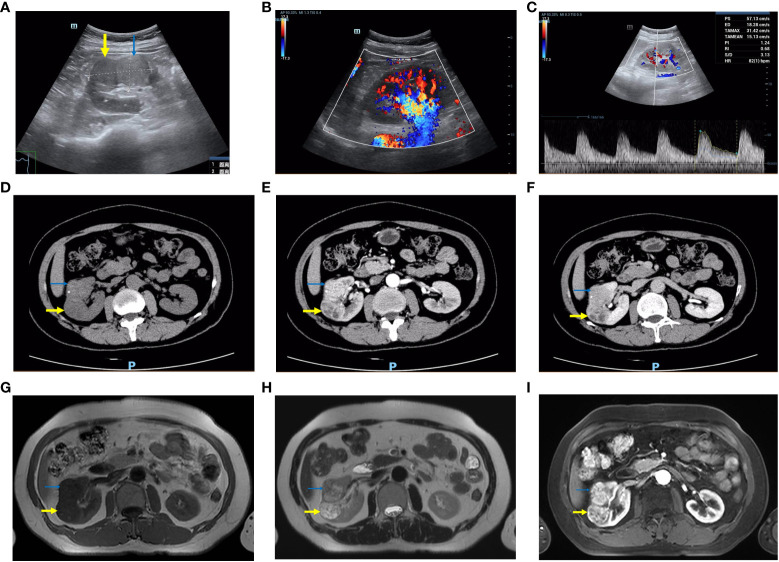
Imaging features of the right renal lesion. The thick yellow arrow is the CCRCC and the thin blue arrow is the CCPRCT. (A–C) Color ultrasound. (A) The boundary between the two masses is clear. (B) The blood flow signals were abundant. (C) High-speed arterial spectrum. (D–F) CT. The density of the CCRCC was lower than that of the CCPRCT in plain scan, and the CCPRCT had some calcifications. Both masses were obviously enhanced in the arterial phase. The CCRCC has cystic areas. The enhancement degree of the two masses decreased during excretion. (G–I) MRI. (G) T1WI (H) T2WI (I) Contrast-enhanced scan.
